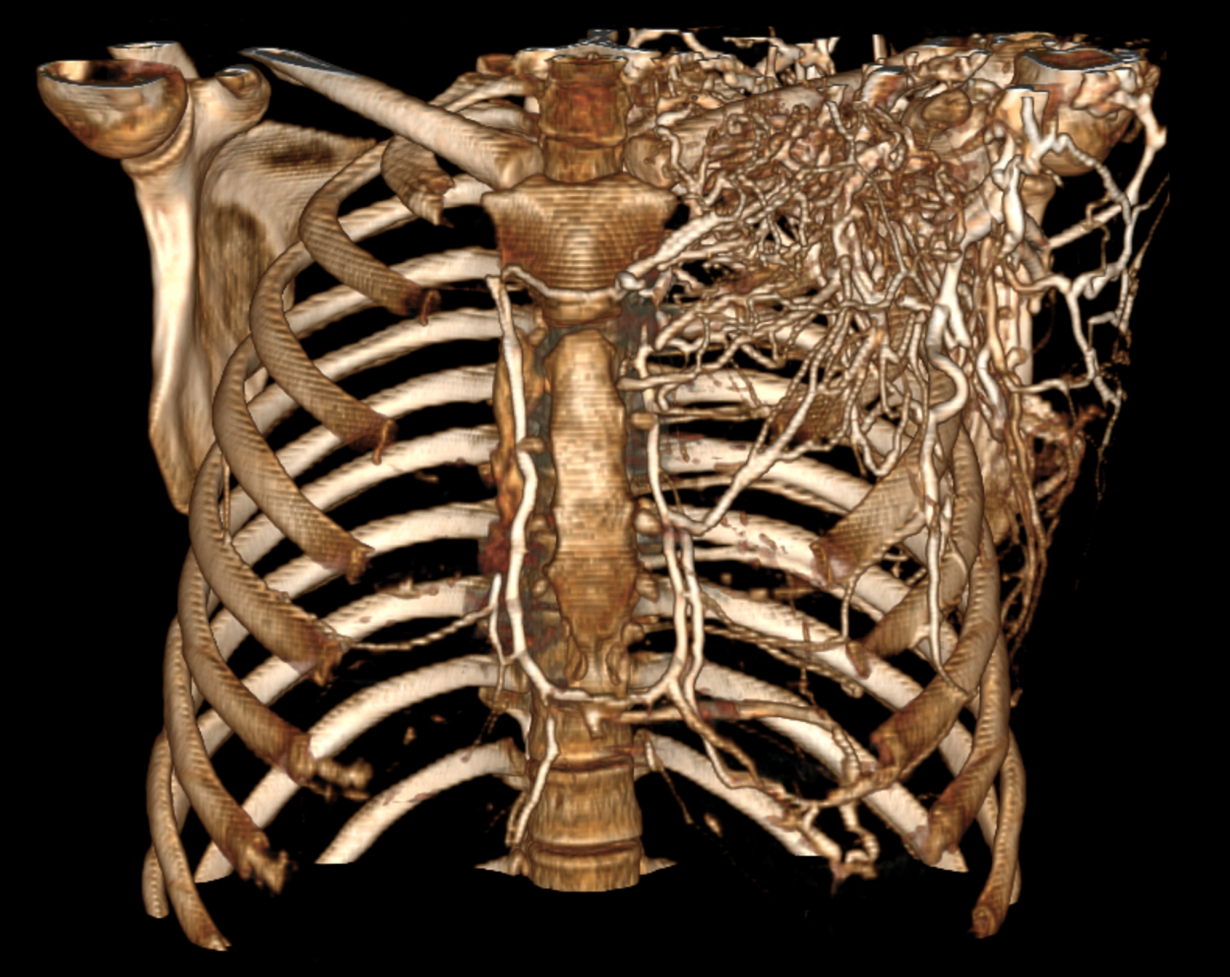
Three-dimensional reconstruction of the contrast-enhanced chest CT Three-dimensional reconstruction of the contrast-enhanced chest CT, showing venous ectasia and contrast enhancement in the venous system of the left upper limb.
